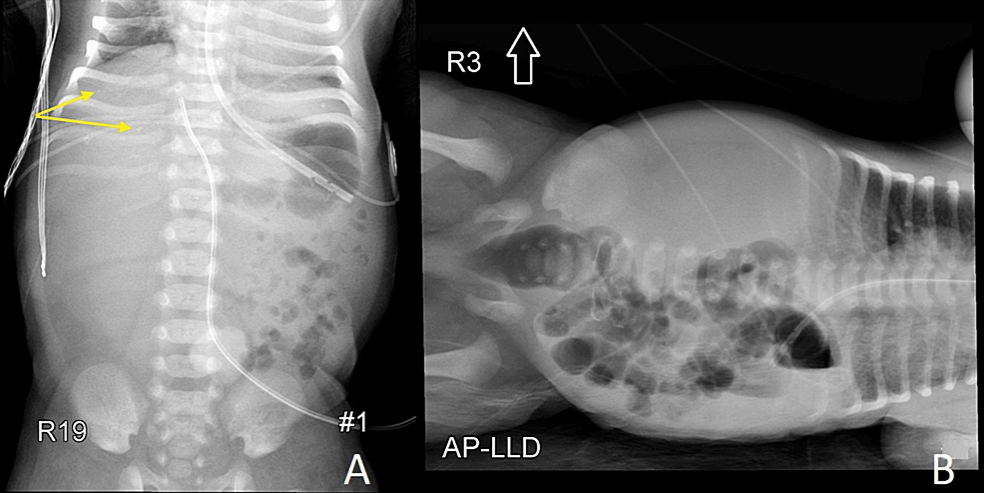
Anteroposterior (A) and left lateral decubitus (B) radiographic views of the abdomen. The images demonstrate absence of gas in the right hemiabdomen and a few small coarse calcifications predominantly in the upper abdomen (yellow arrows; A). There is a mass effect causing deviation of the UVC catheter to the left as well as displacement of the bowel loops to the left. AP-LLD: anteroposterior left lateral decubitus
